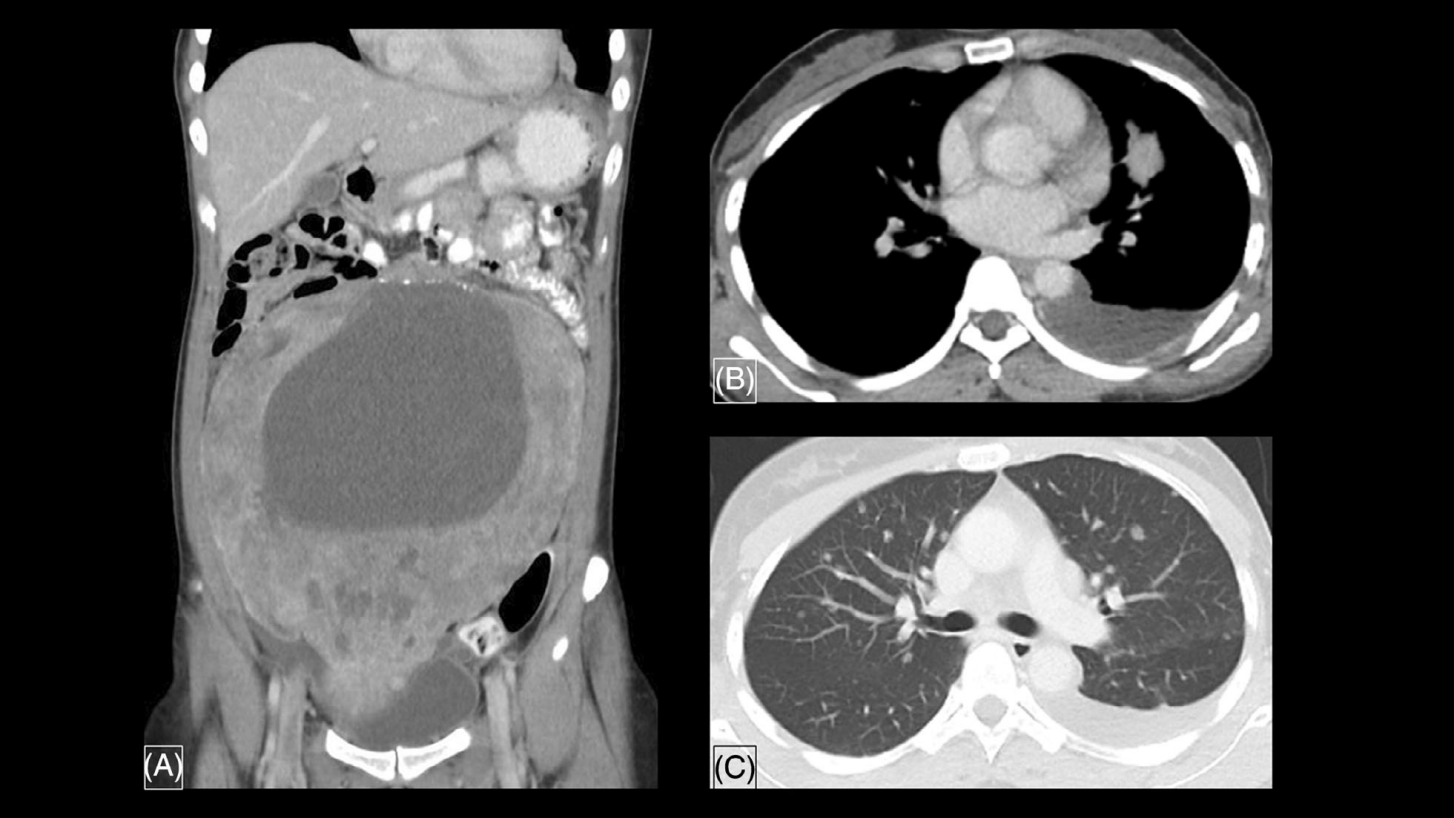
A contrast‐enhanced CT scan of the abdomen showing a large solid cystic mass arising from the pelvis occupying almost the entire lower abdomen, minimal free fluid is seen. (A) A solid irregular lung lesion is seen at the left upper lobe (B). HPE proven lung adenocarcinoma with ipsilateral pleural effusion with multiple bilateral small lung metastases (C)
